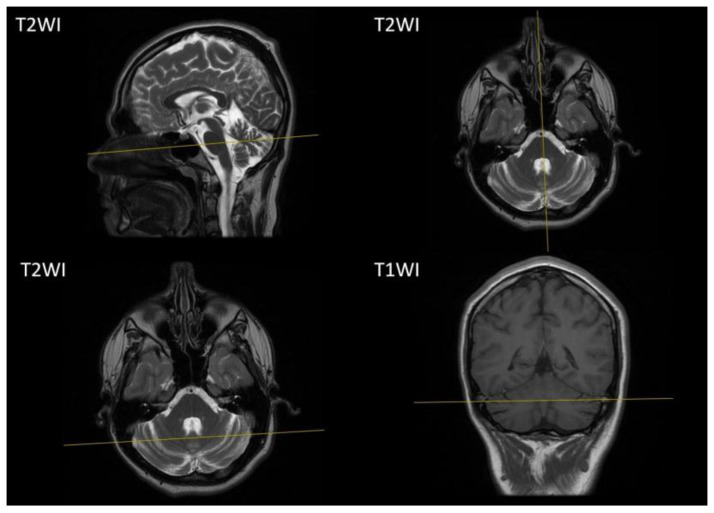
Brain MRI of our proband. Sagittal, axial T1-weighted images and coronal T2-weighted images demonstrate atrophic changes of the cerebellum. T1WI, T1-weighted image; T2WI T2-weighted image.
